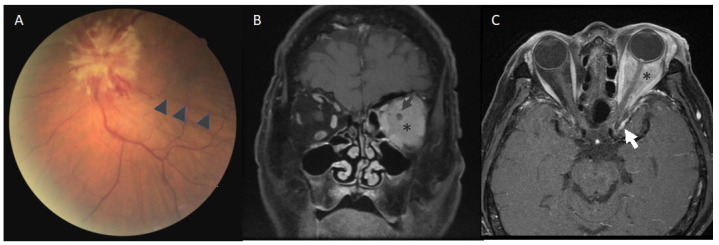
(A) Fundoscopy of the left eye showed a prominent swollen disc, retinal venous dilatation, and chorioretinal folds (arrowhead). (B) Coronal magnetic resonance imaging (MRI) (T1W1) demonstrated the left retrobulbar mass (aristek) encasing the optic nerve (arrow). (C) Axial MRI (T1W1) showed a homogenous lesion (aristek) extending from the left peribulbar space to the cavernous sinus (arrow).
